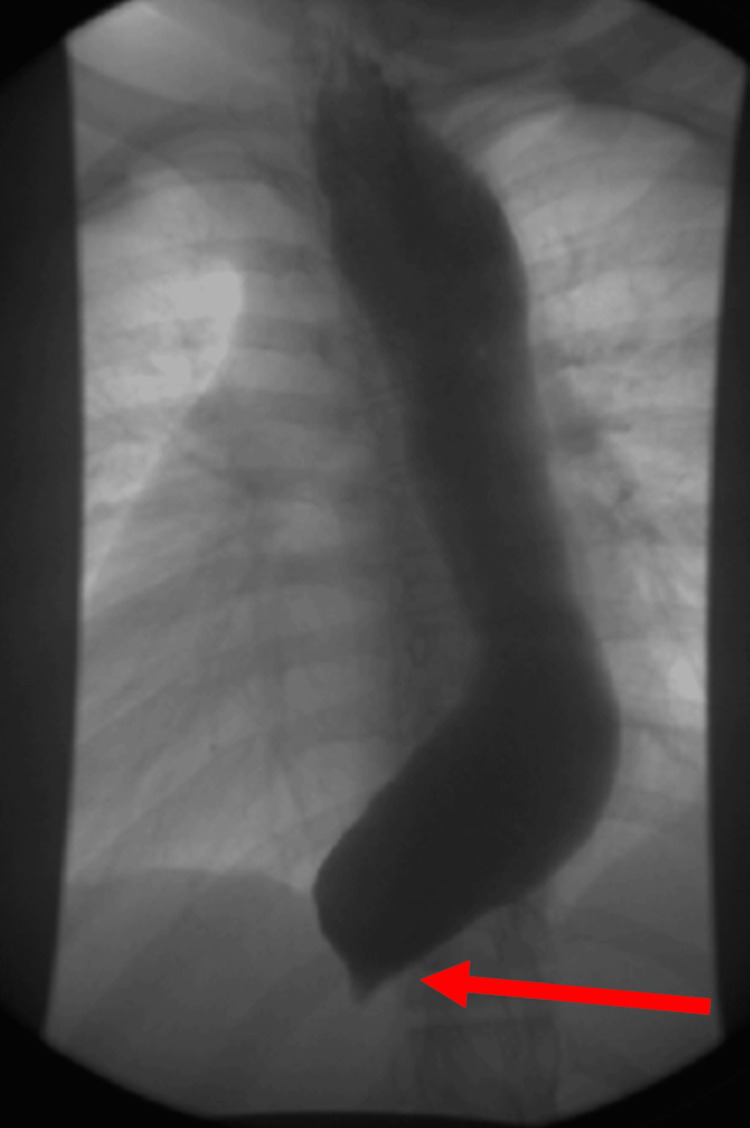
X-ray Esophagogram with Contrast The red arrow points to the contrast failing to progress past the gastroesophageal junction, creating tapering near the gastroesophageal junction
